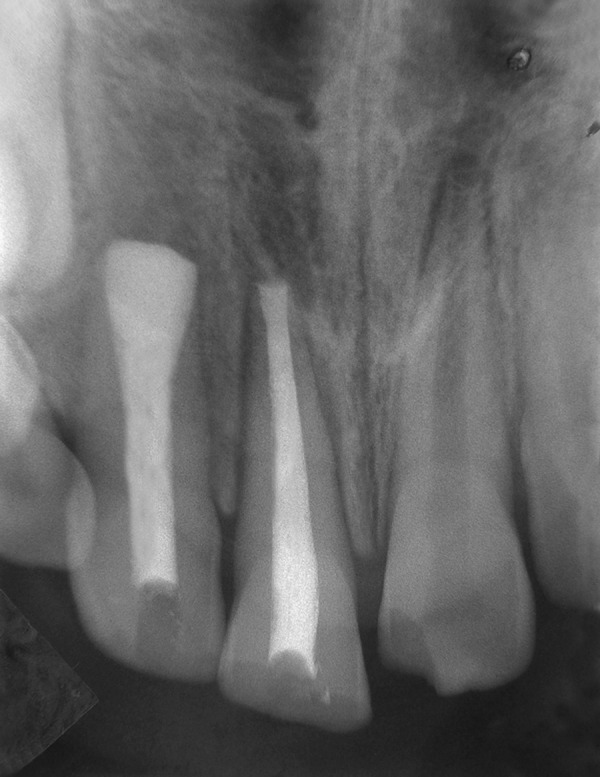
Two years follow-up intraoral radiograph
